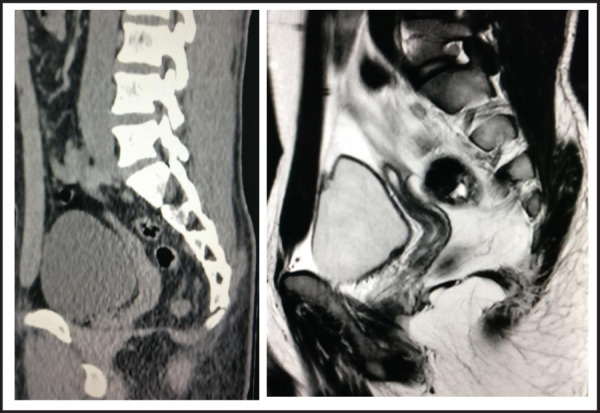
Sagittal image CT scan and MRI T2 WI: Uterus is seen posterior to bladder. The uterus shows hyperintense fluid structure in the endometrial cavity and hypointense junctional line.
